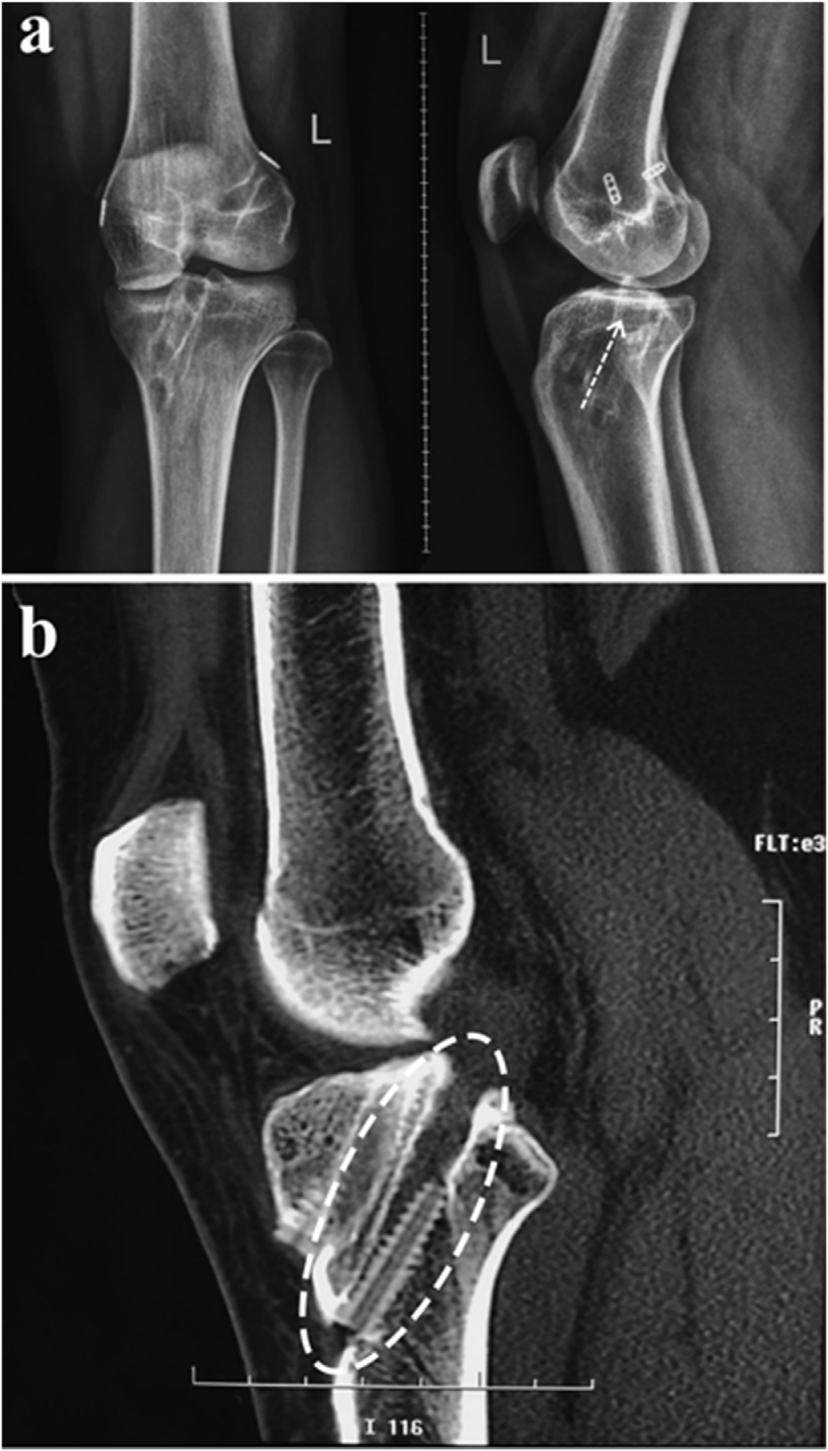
Post-PCL-reconstruction.(a) Plain X-ray showed that angel and direction of tibial tunnel was too anterior (white arrow) and (b) 3D-CT scan of left knee showed that tibial tunnel for PCL was too anterior than normal footprint (white circle).
